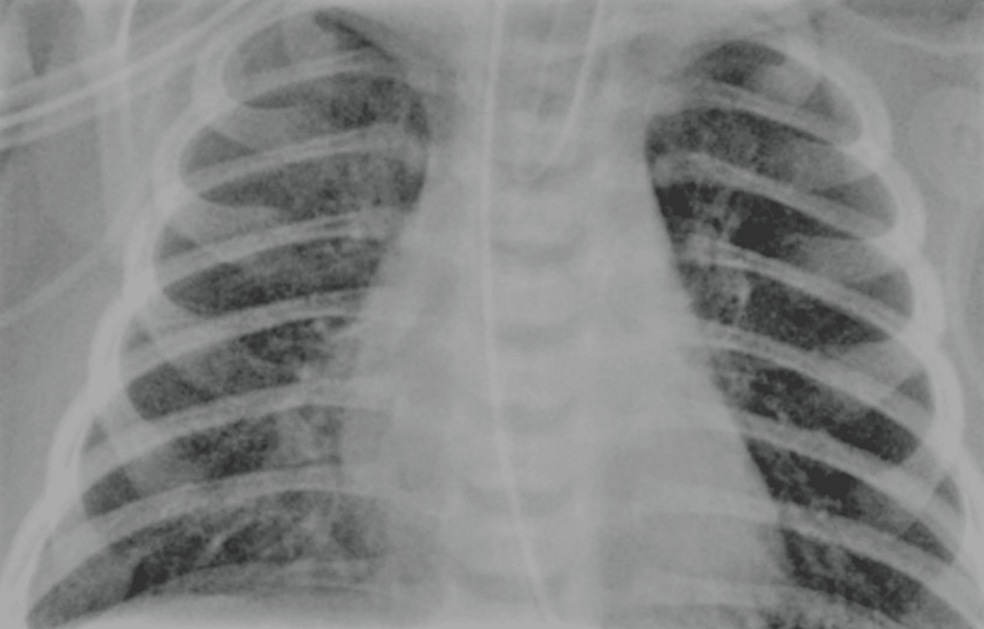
Chest X-ray showing pneumonia.
